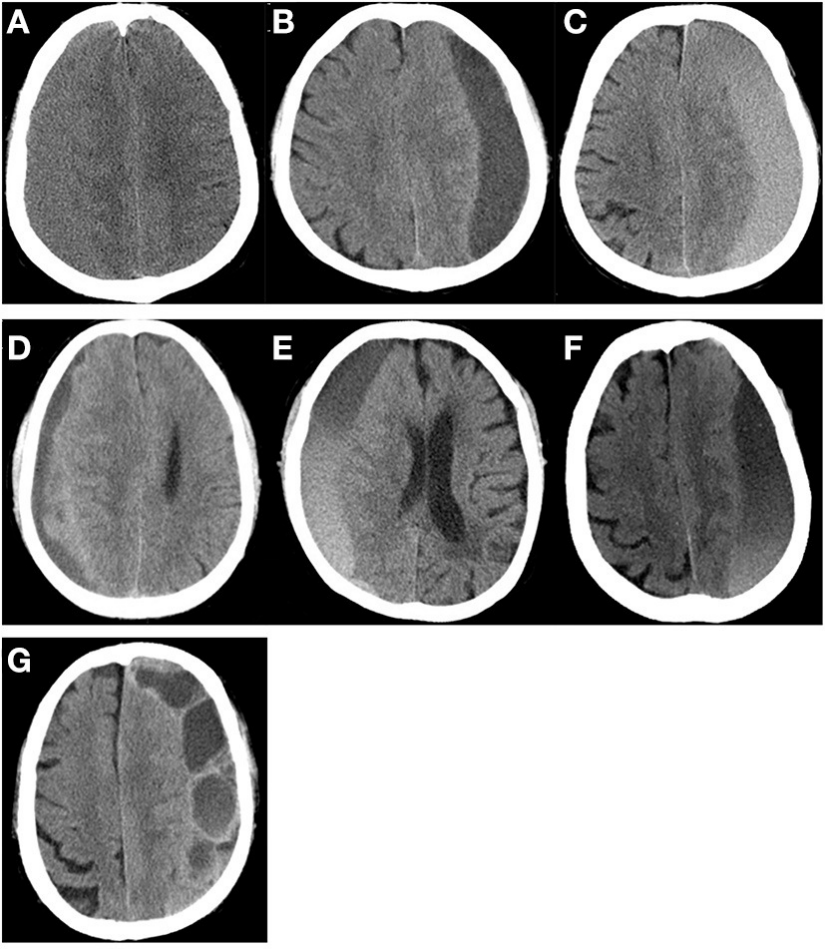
CT density classification of patients with chronic subdural hematoma. (A) isodense subtype, (B) hypodense subtype, (C) hyperdense subtype, (D) laminar type, (E) separated type, (F) gradation subtype, (G) trabecular type.
